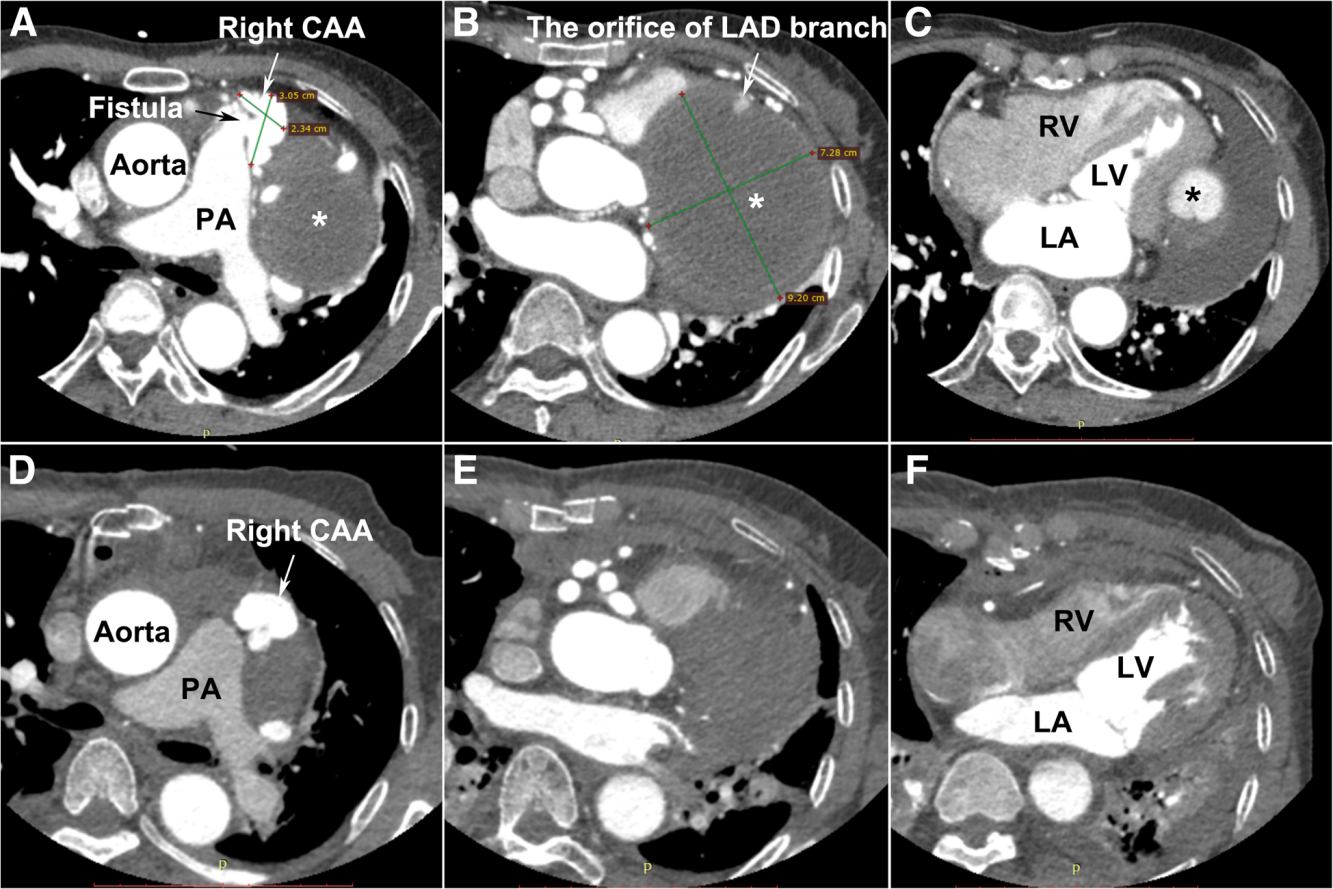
The transverse view of coronary CTA: a Giant right CAA with RCA-PA fistula; b and c left CAA, compressing the LV wall; d, e and f Postoperative CTA demonstrated no RCA-to-PA shunting and the oppression of the giant left CAA to left ventricle had been released. The diameter of left CAA decreased significantly. * CAA, coronary artery aneurysm; PA, pulmonary artery; LAD, left anterior descending coronary artery; LV, left ventricle; RV, right ventricle; CTA, computed tomography angiography
